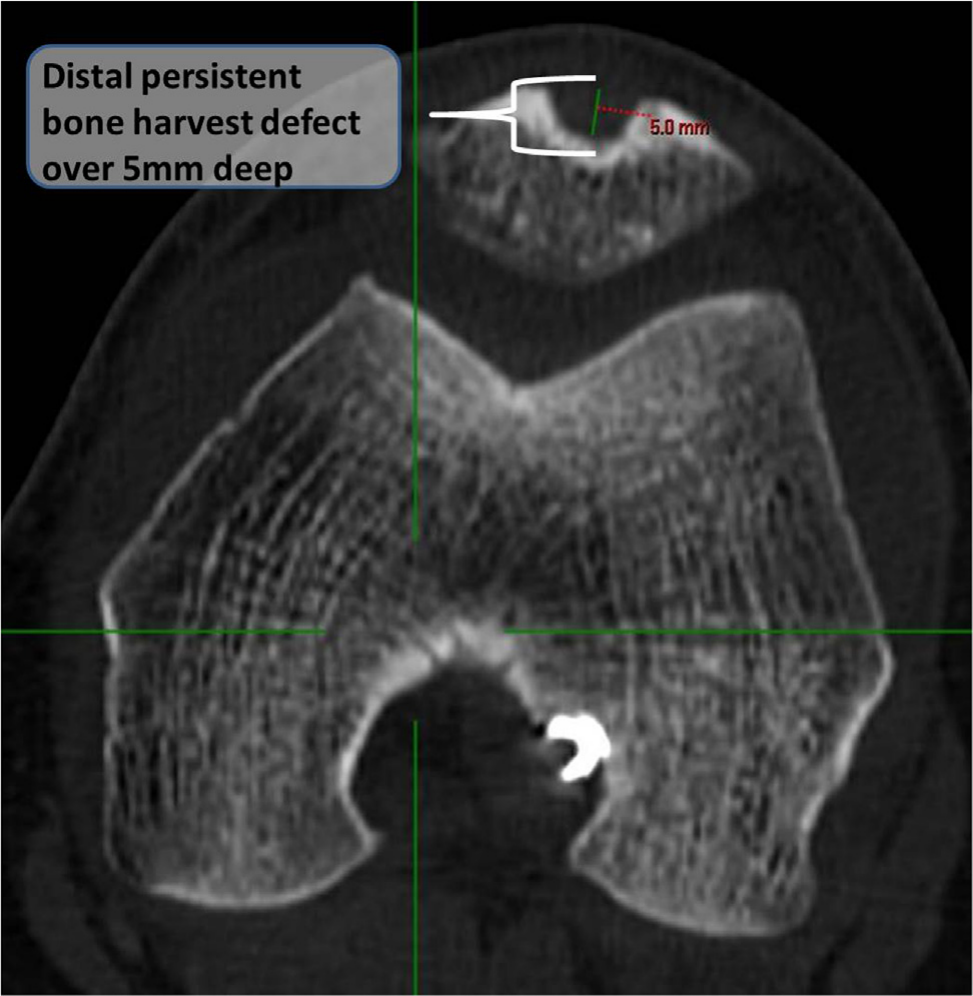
Two-dimensional axial computed tomography cut of a left knee showing over a 5-mm deep persistent distal patella bone defect, implying only partial fill of the harvest site after the primary surgery.4.On the sagittal CT cut through the center of the distal bone defect, measurements are performed for planning harvesting a 15- to 18-mm-long proximal patellar bone plug and ensuring an 8- to 10-mm “safety” bone bridge remaining between the distal and proximal bone plug harvest sites (Fig 4).
